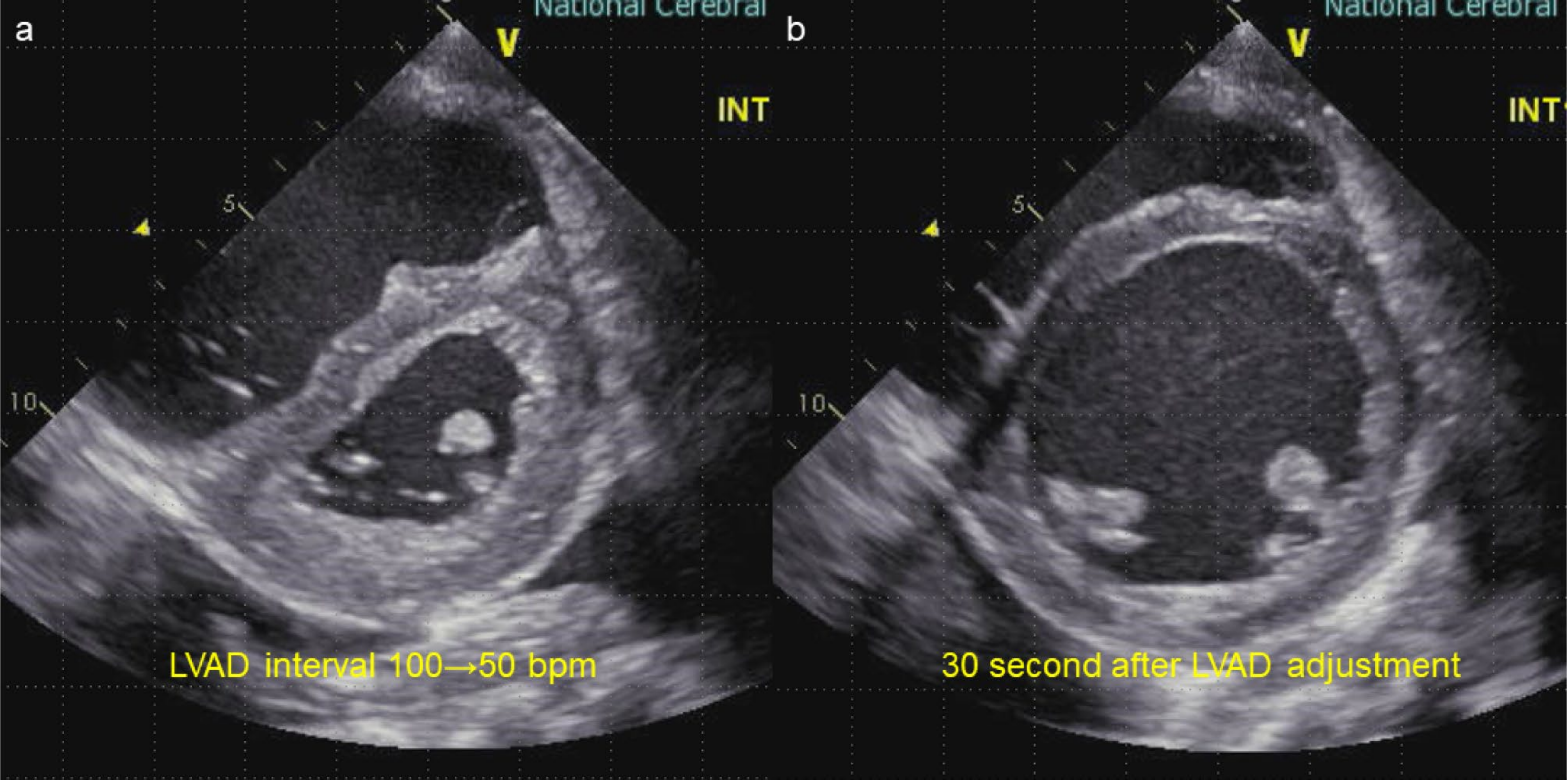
Echocardiographic findings during left ventricular assist device pump flow adjustment. (a, b): This echocardiographic observation showed dramatic hemodynamic changes; the D-shape of the left ventricle recovered to an O-shape within 30 s
